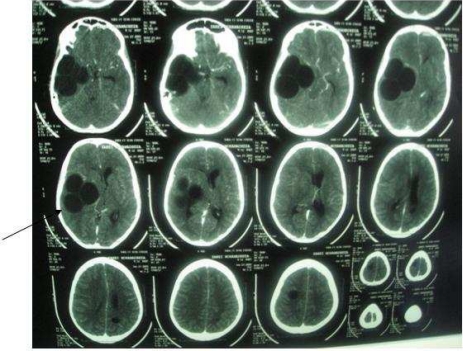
Brain computed tomography scan shows large multilocular cystic lesions about 70×65 mm in right temporal lobe with some mass effect over the adjacent structures.
